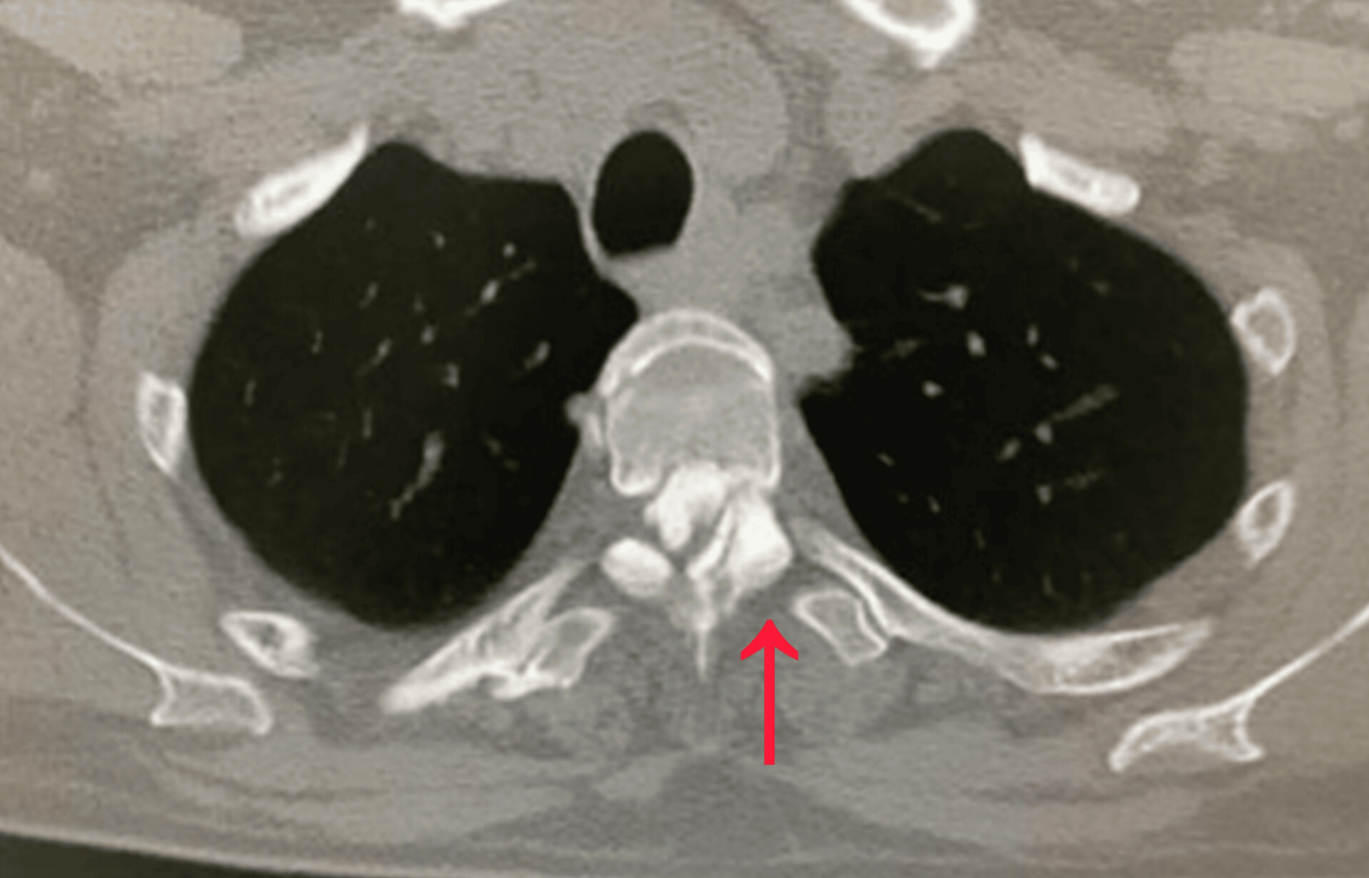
Pre-operative computed tomography (CT) demonstrates circumferential spinal canal compromise at the T3-T4 level due to anterior OPLL and posterior OYL (red arrow). OPLL: ossified posterior longitudinal ligament; OYL: ossified yellow ligament
